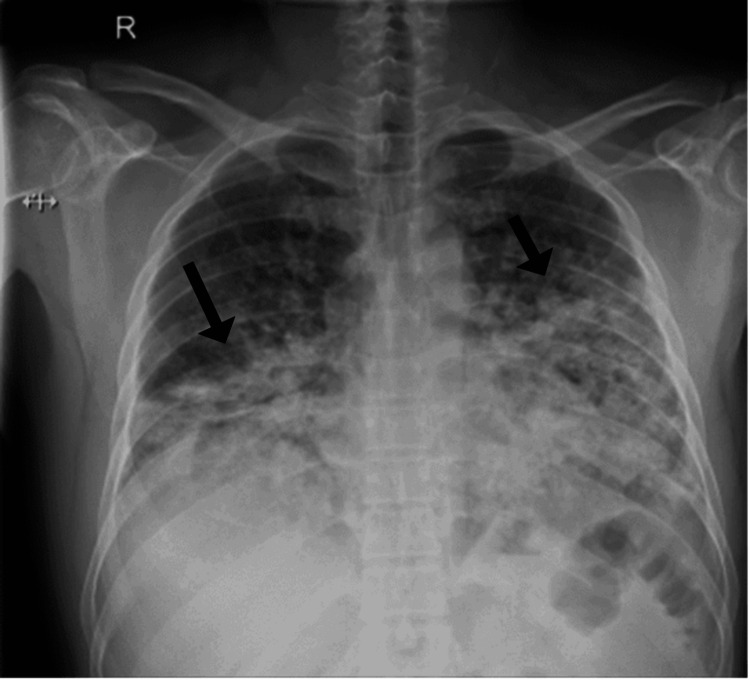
Chest radiography revealed bilateral heterogeneous opacities in the lower lung zones, suggestive of an airspace disease process (black arrows).
